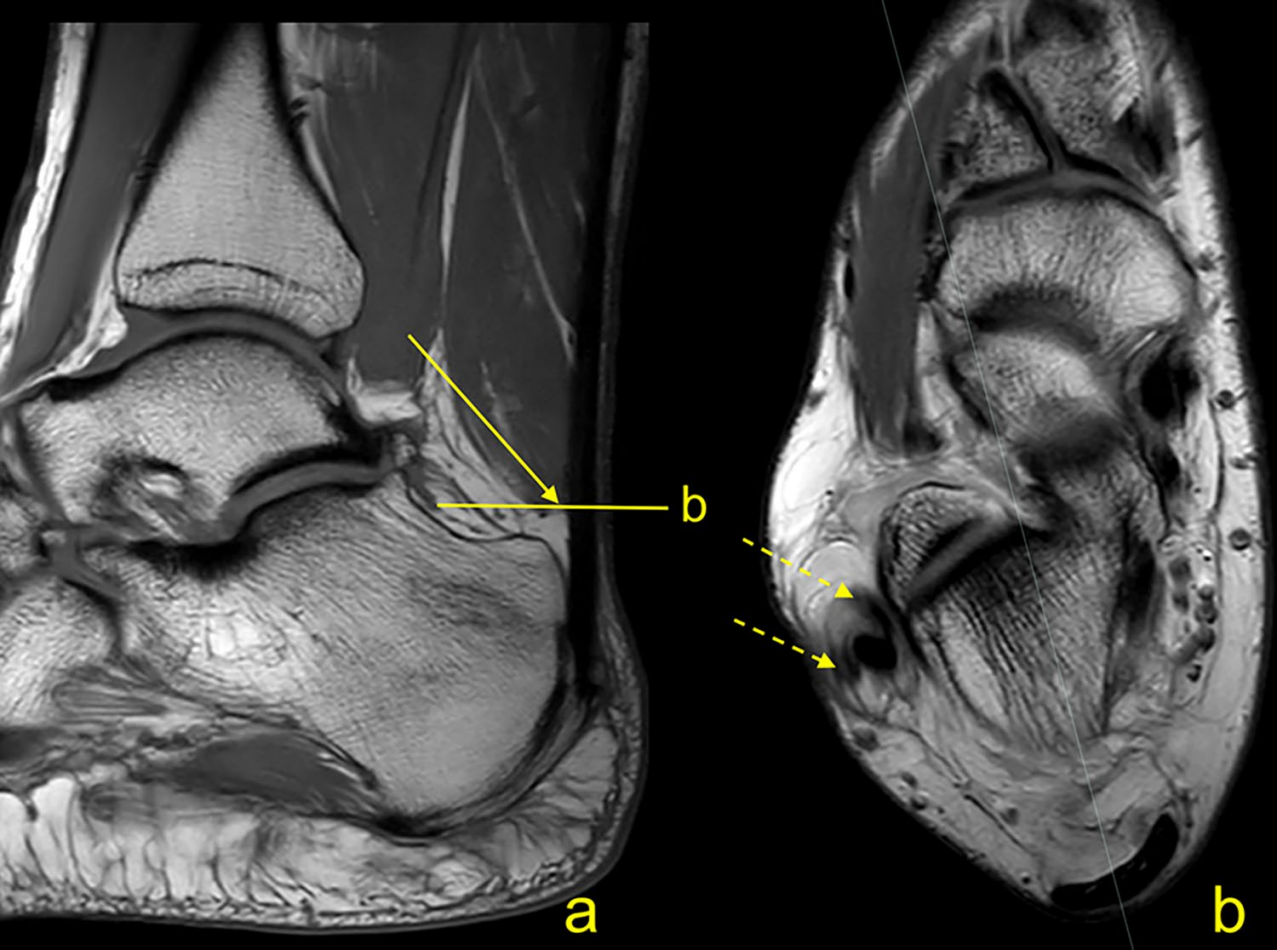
A 31-year-old female with chronic lateral ankle pain, normal Achilles tendon and short free tendinous part measuring 8 mm. MRI (a T1–w TSE (turbo spin echo), b PD–w TSE) showed the normal Achilles tendon and split rupture of the peroneus brevis as the cause of symptoms (dashed arrows). The lowest level where the soleus muscle fibers can be identified is marked with an arrow (a)
